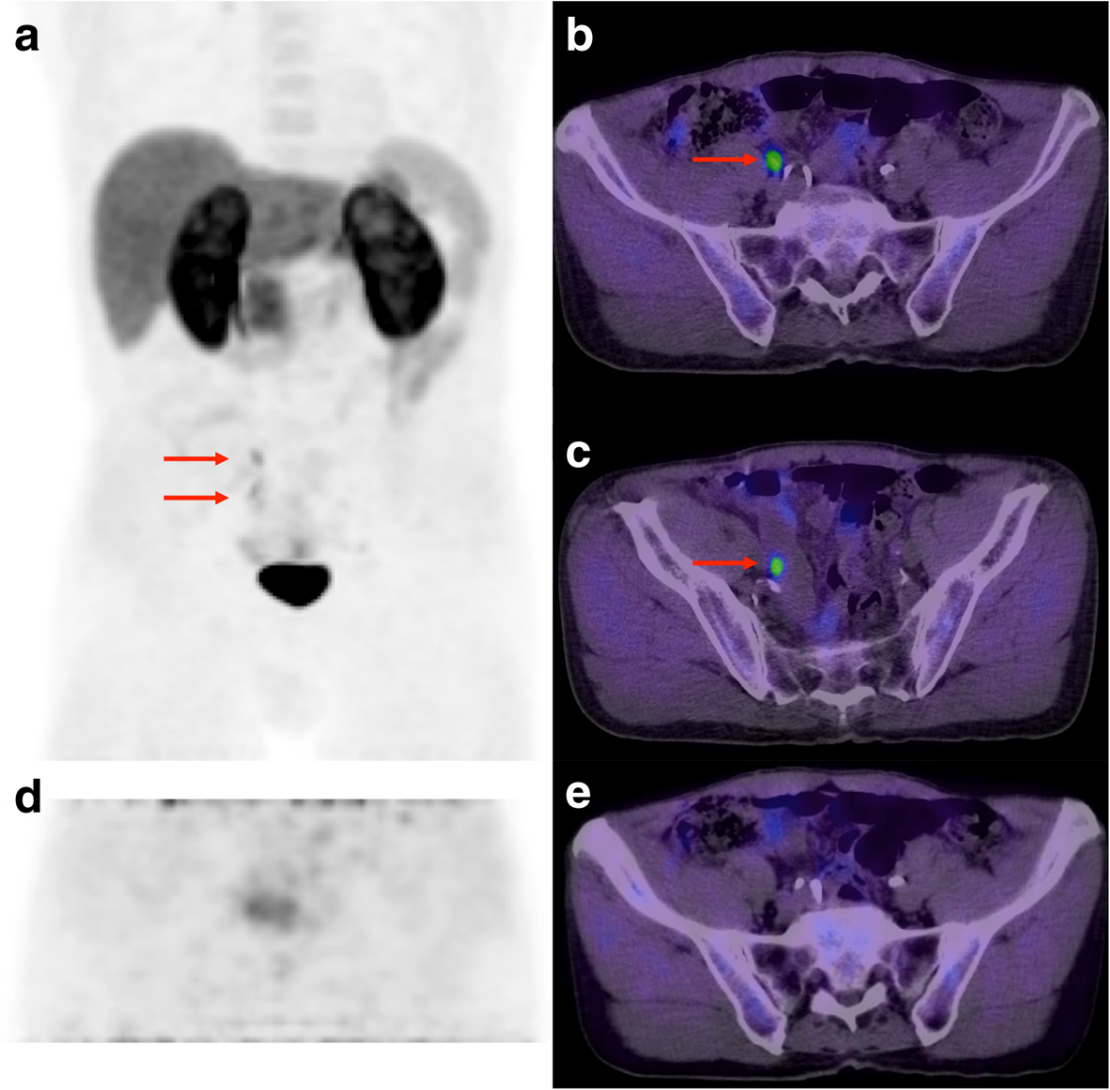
Restaging FCH-PET/CT in a 68 y.o. patient with biochemical relapse following radical prostatectomy. Initially, PCa Gleason sum was 7. PSA was 0.35 mg/L at time of examination. Whole-body MIP (a) and transaxial fusion slices (b, c) showed two foci of moderate uptake in the right iliac nodal area (red arrows) that were initially perceived as suspicious for nodal relapse. However, dynamic PET MIP (d) and fusion series (e) showed no early focal activity at either site, which was consistent with urinary activity on the whole-body scan. This study was interpreted as negative and the patient underwent salvage radiotherapy
